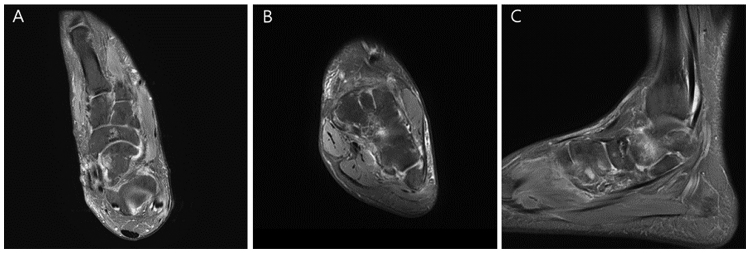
Postoperative T2 magnetic resonance imaging of the left ankle. (A) T2 axial view, (B) T1 coronal view, and (C) sagittal proton density fast spin echo view. These postoperative images demonstrate radiographic evidence of graft incorporation without evidence of displacement, collapse, or graft resorption.
